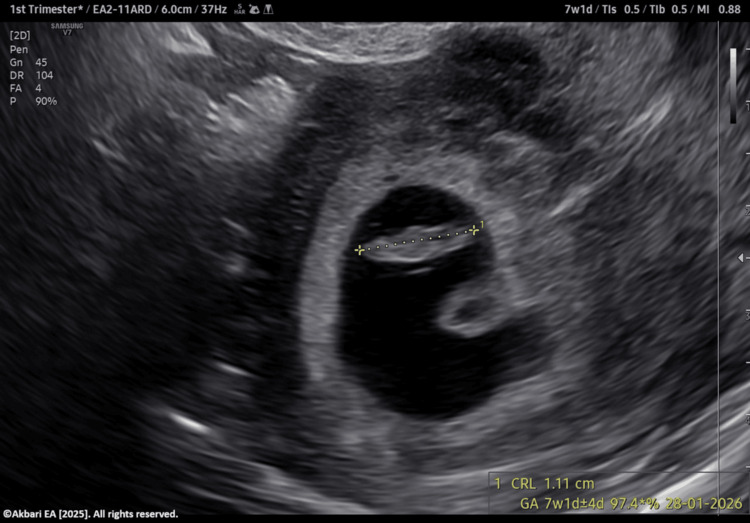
Transvaginal ultrasound image showing crown rump length (CRL) of seven weeks and one day Original image created by the author, ©Akbari EA 2025.
